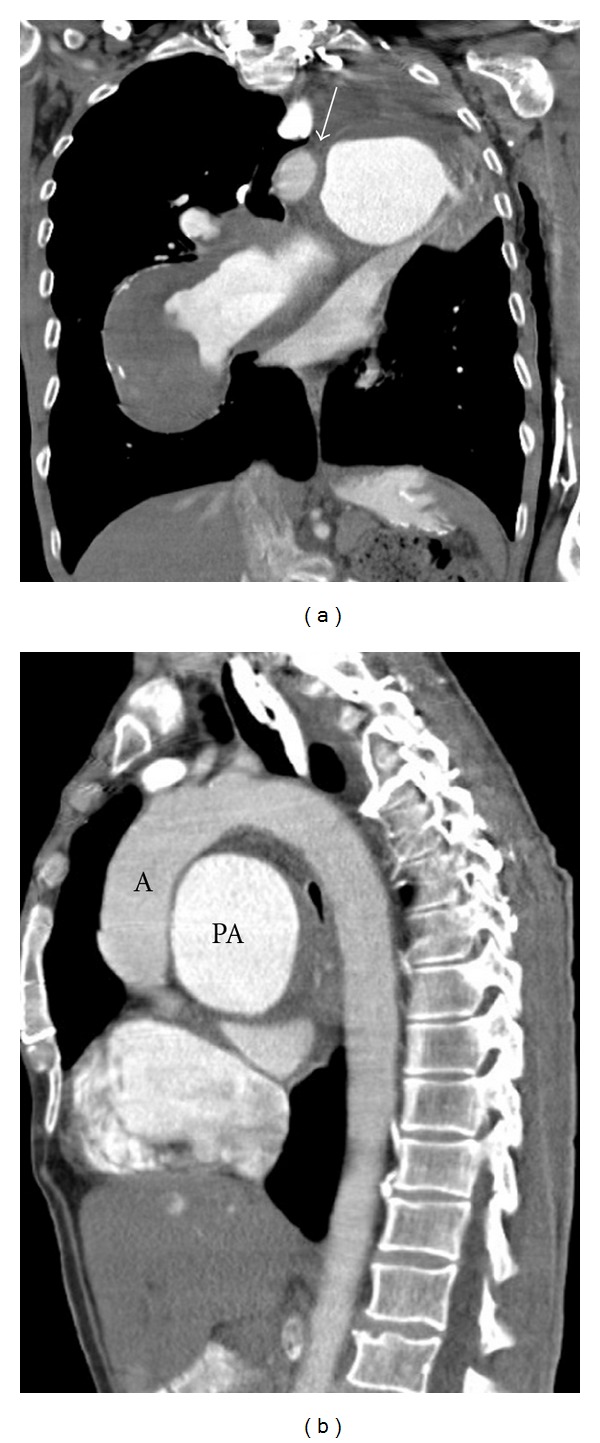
Coronal (a) and sagittal reformat (b) from the CT. The aortopulmonary window (arrow in (a)) is almost completely obstructed by the enlarged left pulmonary artery. (b) The sagittal reformat demonstrates the narrow space between the aorta (A) and pulmonary artery (PA).
